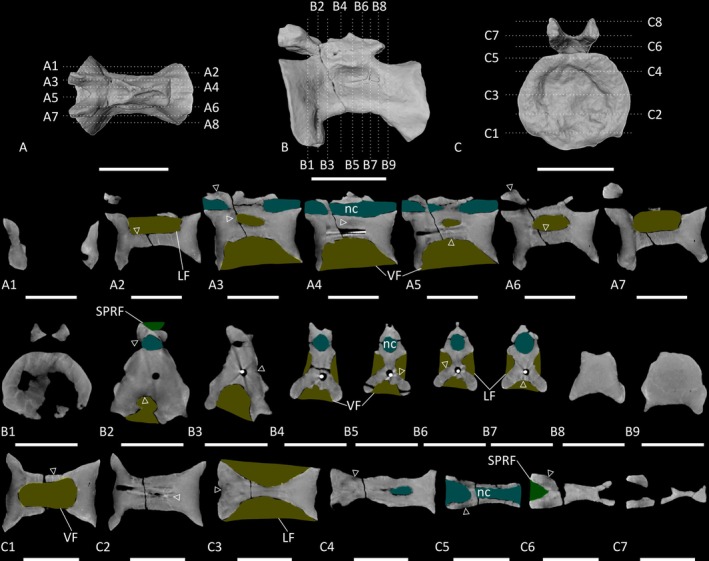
CT scan of *Tornieria africana* posterior caudal vertebra MB.R.2956.12. (A–C) 3D rendering (A) dorsal view, (B) left lateral view, (C) anterior view; (A1–A7) CT scan slices in parasagittal cross‐section, (B1–B9) CT scan slices in transverse cross‐section, (C1–C7) CT scan slices in horizontal cross‐section. LF, lateral fossa; nc, neural canal; SPRF, spinoprezygapophyseal fossa; VF, ventral fossa. Key: Blue highlight, neural canal; green highlight, neural spine fossa; yellow highlight, centrum fossa; white arrow, pneumatic bone texture. Scale bars = 100 mm.
